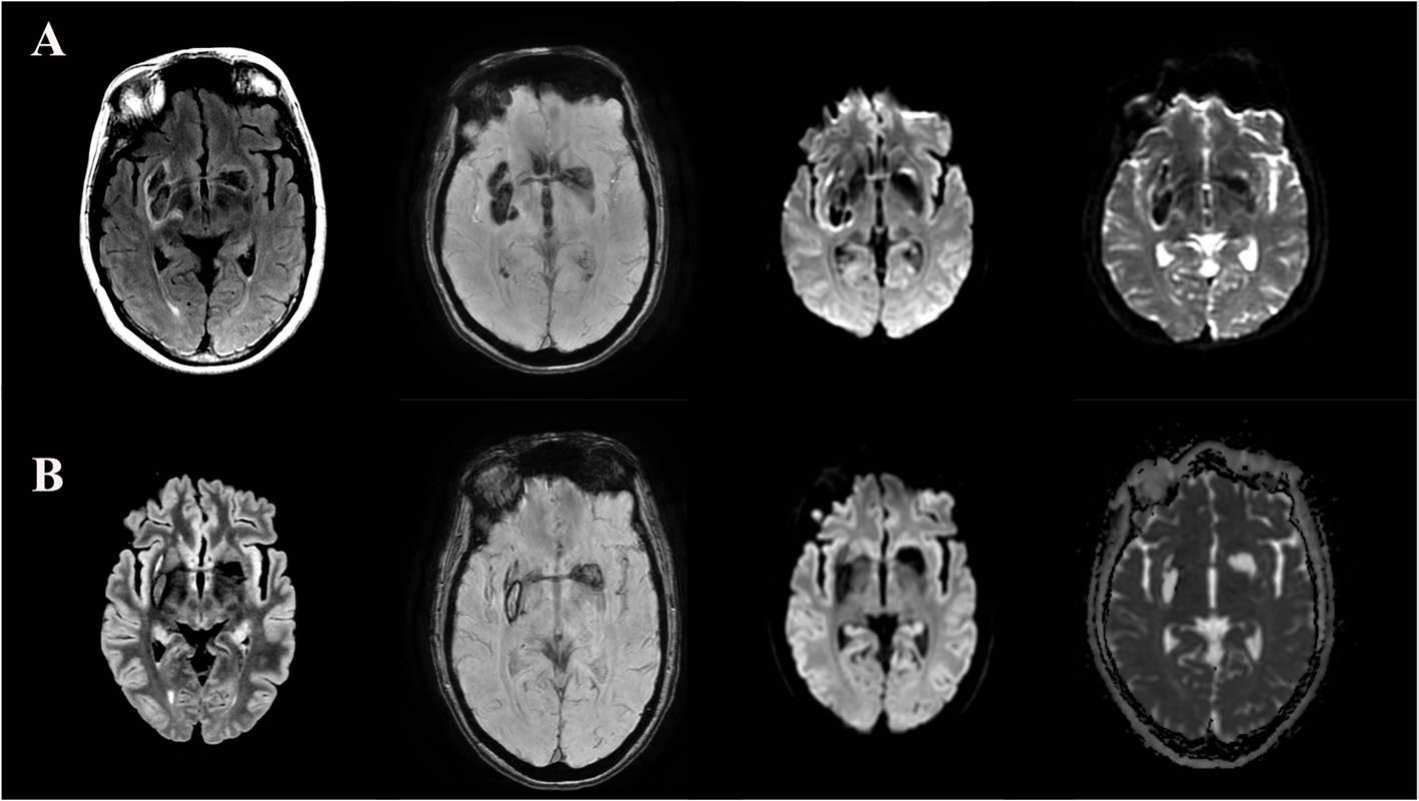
A Initial and (B) 7-month follow-up magnetic resonance images demonstrating the extent of right basal ganglia hemorrhage, contralateral extension and delineation of the AC tracts. At 7 months follow-up, the BGH and intraventricular hemorrhage had resolved, but residual susceptibility signal was appreciated along the extent of hemorrhage. From left to right, sequences presented are: T2 Fluid Attenuated Inversion Recovery (T2 FLAIR), Susceptibility-Weighted Angiography (SWAN), isotropic image/diffusion-weighted image (DWI), and apparent diffusion coefficient (ADC). Images acquired with a General Electric 750W 3 T MRI scanner
